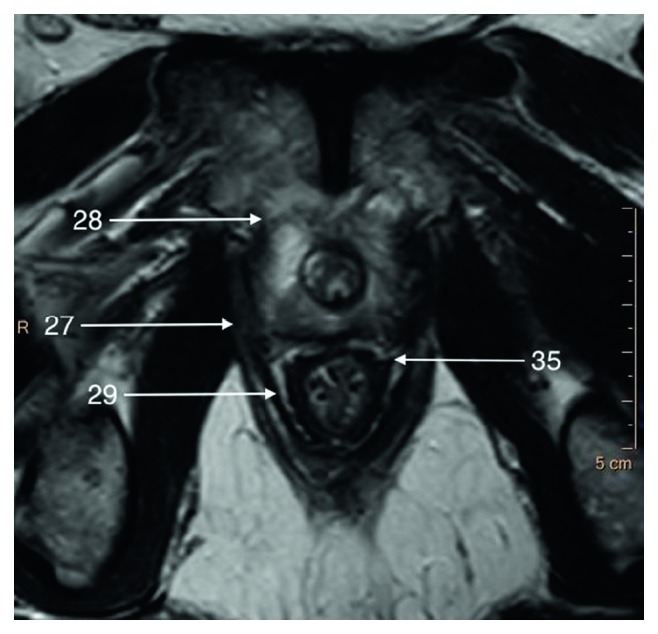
T2-weighted axial image of a border between apical and middle parts of the prostate gland.
